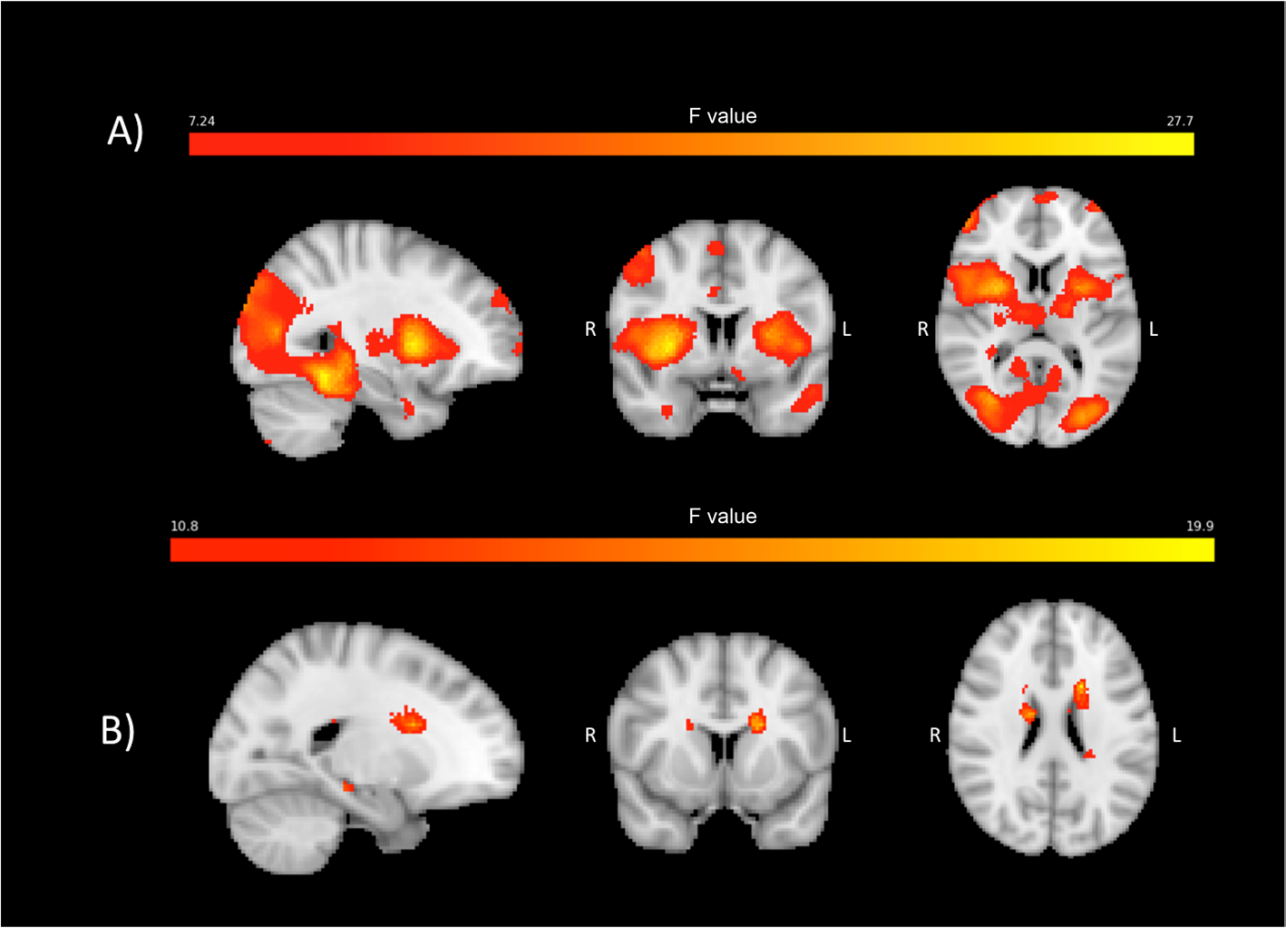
Main effect of painful heat applied to the left forearm at baseline (i.e. pre-treatment). A) immediate BOLD response to painful stimulation (measured starting with the MRI frame during which the painful heat stimulus began and ending 12.5 s later). B) delayed BOLD response to painful stimulation (measured starting 12.5 s after onset of painful heat stimulus and ending 12.5 s later). Hotter colors represent stronger activation
